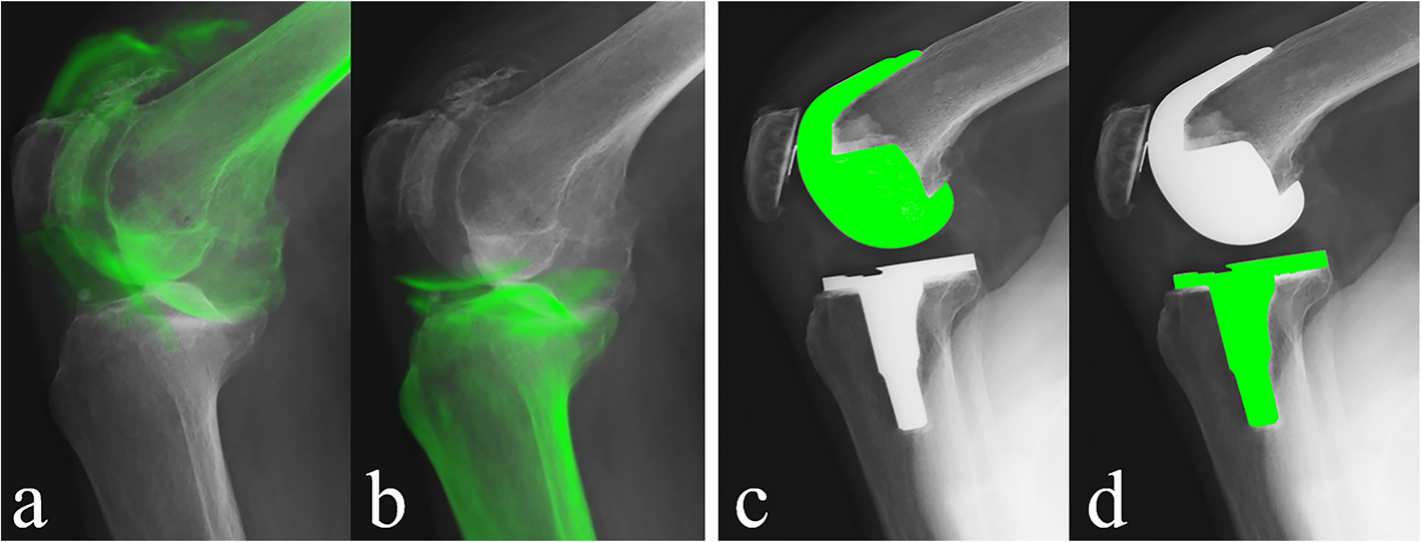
Computed tomography slices were used to create density-based digitally reconstructed radiographs (DDR) of the femur (a) and the tibia (b), which were projected onto the radiographic images of the preoperative knee. Computer-aided design data of the femoral (c) and tibial (d) components were also projected onto radiographic images of postoperative knee
